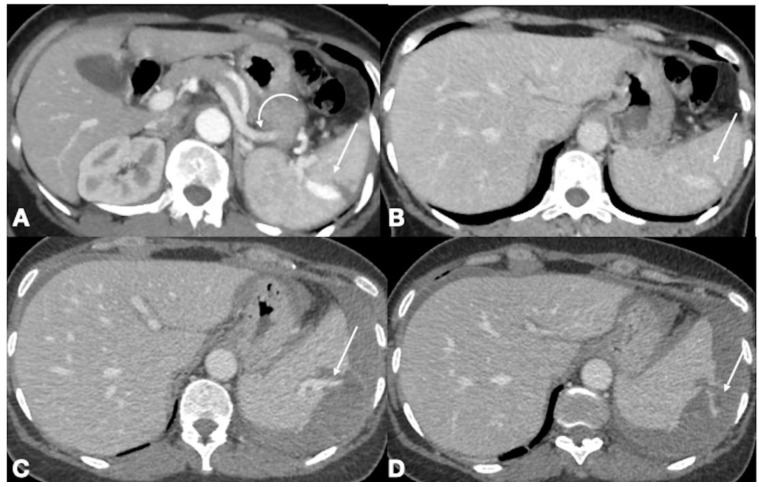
Enhanced-CT acquired in a 43-year old male who sustained major trauma (motor vehicle accident). Admission CT acquired in arterial (A) and portal venous (B) phases shows the presence of a contained vascular injury (pseudoaneurysm) within the splenic laceration (A, arrow). The pseudoaneurysm is associated with an arterio-venous fistula, demonstrated by early opacification of the splenic vein (curved arrow) in the arterial phase, synchronous with that of the splenic artery. The pseudoaneurysm is faintly seen in the following portal venous phase (B), and the arterio-venous fistula is not identifiable in this phase. The patient was scheduled for angiography and embolization but whilst awaiting the procedure, the vascular injury caused a spontaneous splenic rupture with active extrasplenic bleeding (C, arrow) which increased in the subsequent phase (D, arrow). Figure 4A was presented in the poster C-12530 Splenic Emergencies: value of US exploration for the diagnosis at ECR 2020.
